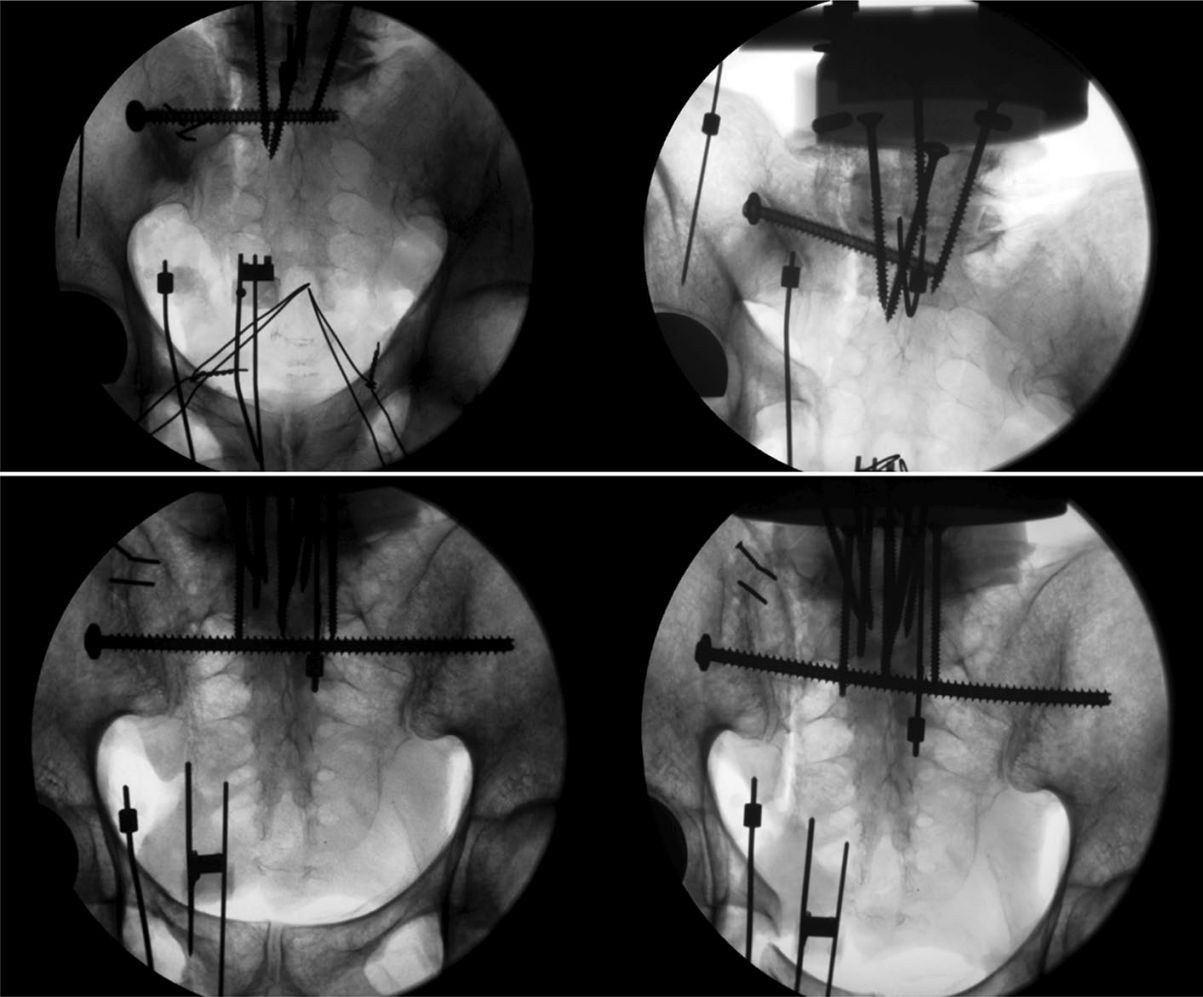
Exemplified anterior–posterior radiographs of a construct instrumented with and SI-Screw (upper) and TITS-Screw (lower), shown at test start (left), and after 15,000 cycles (right)
